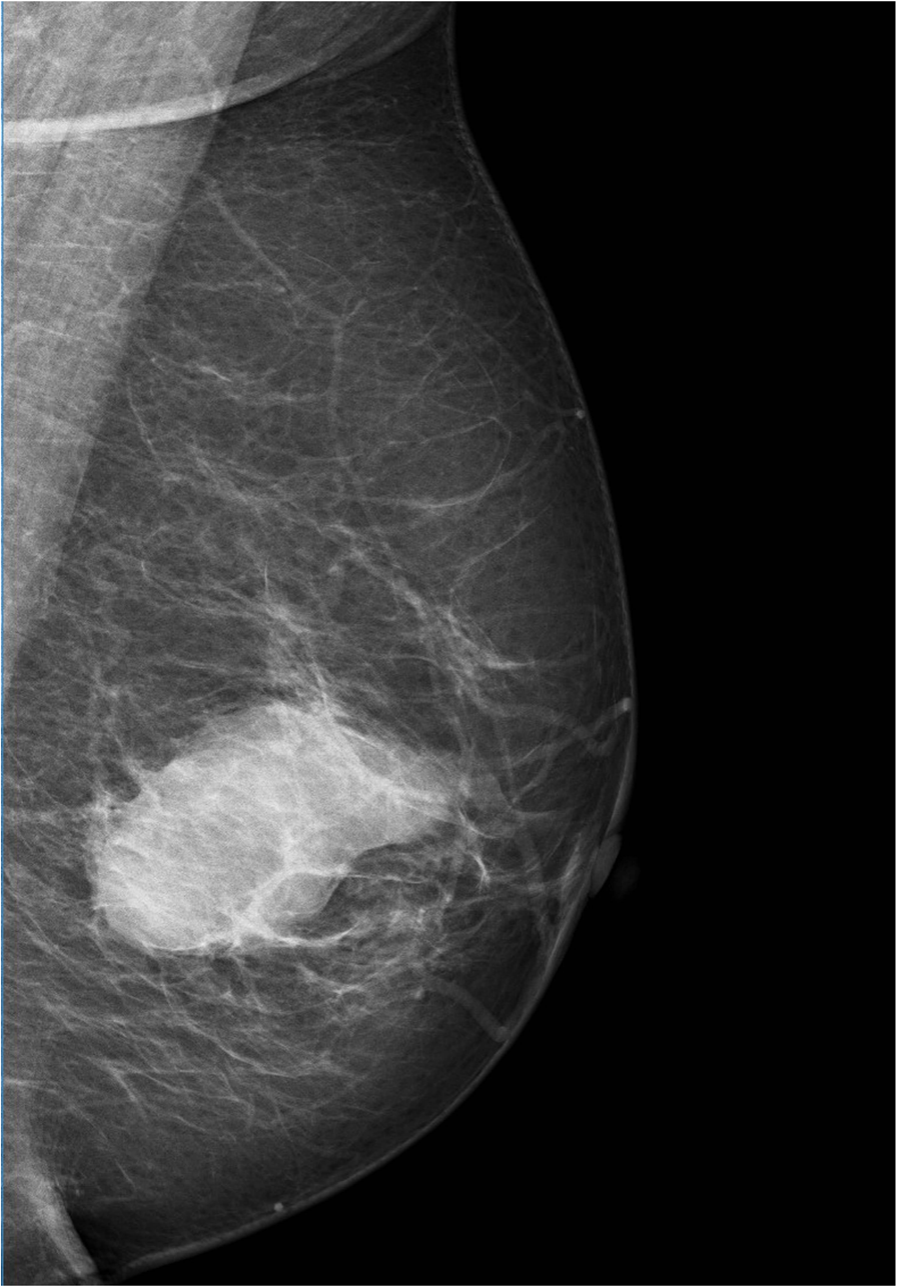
X-ray revealed osteolytic damage and cortex destruction of the left distal femur (a). T2-weighted MRI revealed a heterogeneous high signal mass (b). Gadolinium-enhanced T1-weighted fat-suppression images revealed rim enhancement (c)
